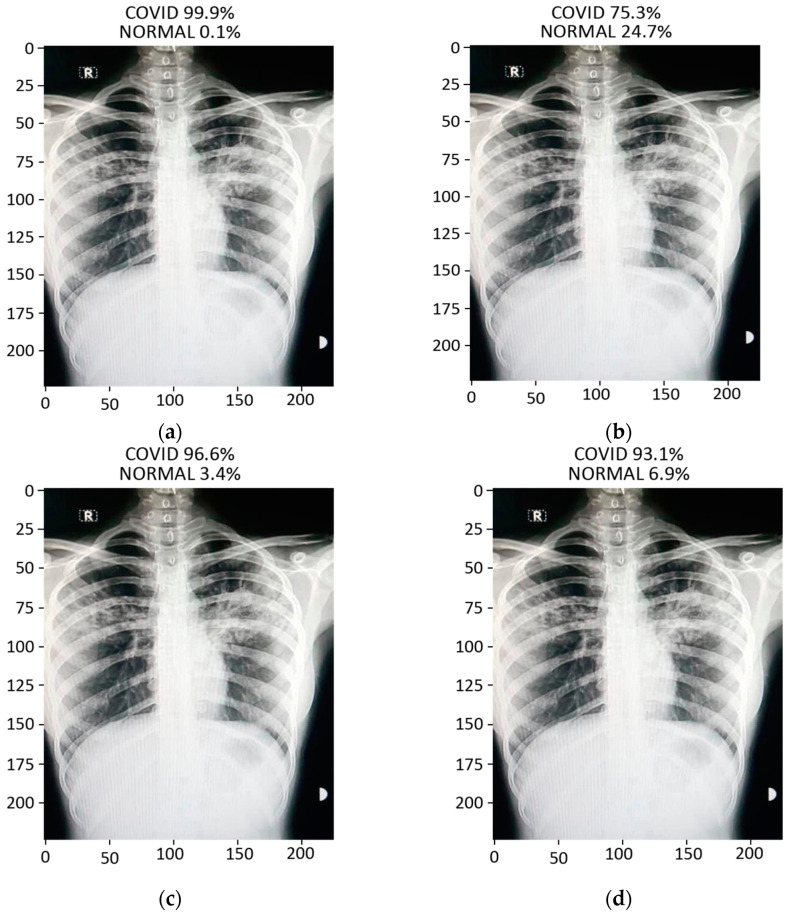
Results of prediction on a verified local Pakistani COVID-19 chest X-ray: (a) DenseNet-121; (b) VGG16; (c) MobileNet; (d) ResNet-50.
